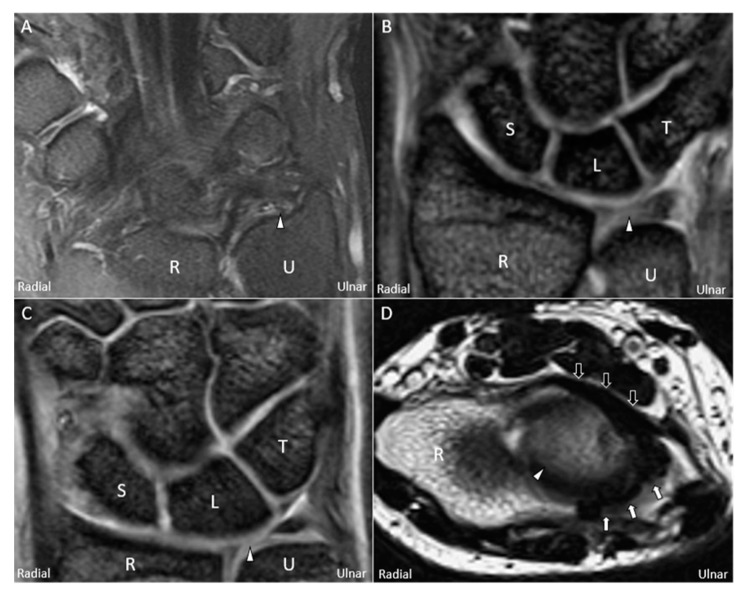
Magnetic resonance imaging (coronal T2-weighted view) showing (A) avulsion of the articular disc *(white arrowhead)* from the ulnar fovea, (B) central tear of the disc, and (C) radial avulsion of the articular disc. (D) Magnetic resonance imaging (axial T2-weighted view) showing a swollen palmar radioulnar ligament *(black arrow)* and a torn dorsal radioulnar ligament *(white arrow)* surrounding the articular disc. U: ulna; R: radius; L: lunate; T: triquetrum; S: scaphoid.
